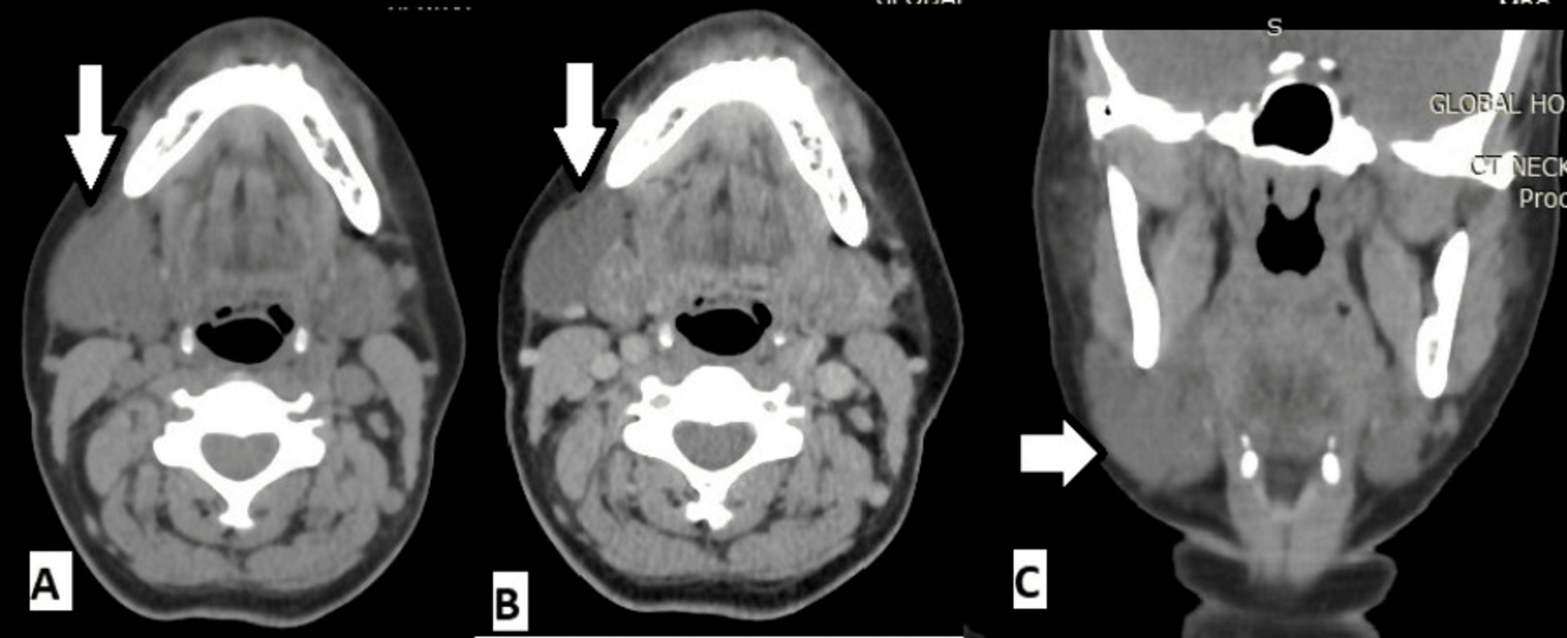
Contrast-enhanced computed tomography showing cystic lesion in the right submandibular region. (A) and (B) Axial cuts showing cystic lesion in the right submandibular lesion marked with the white solid arrow. (C) Coronal cut showing cystic lesion in the right submandibular lesion marked with the white solid arrow.
